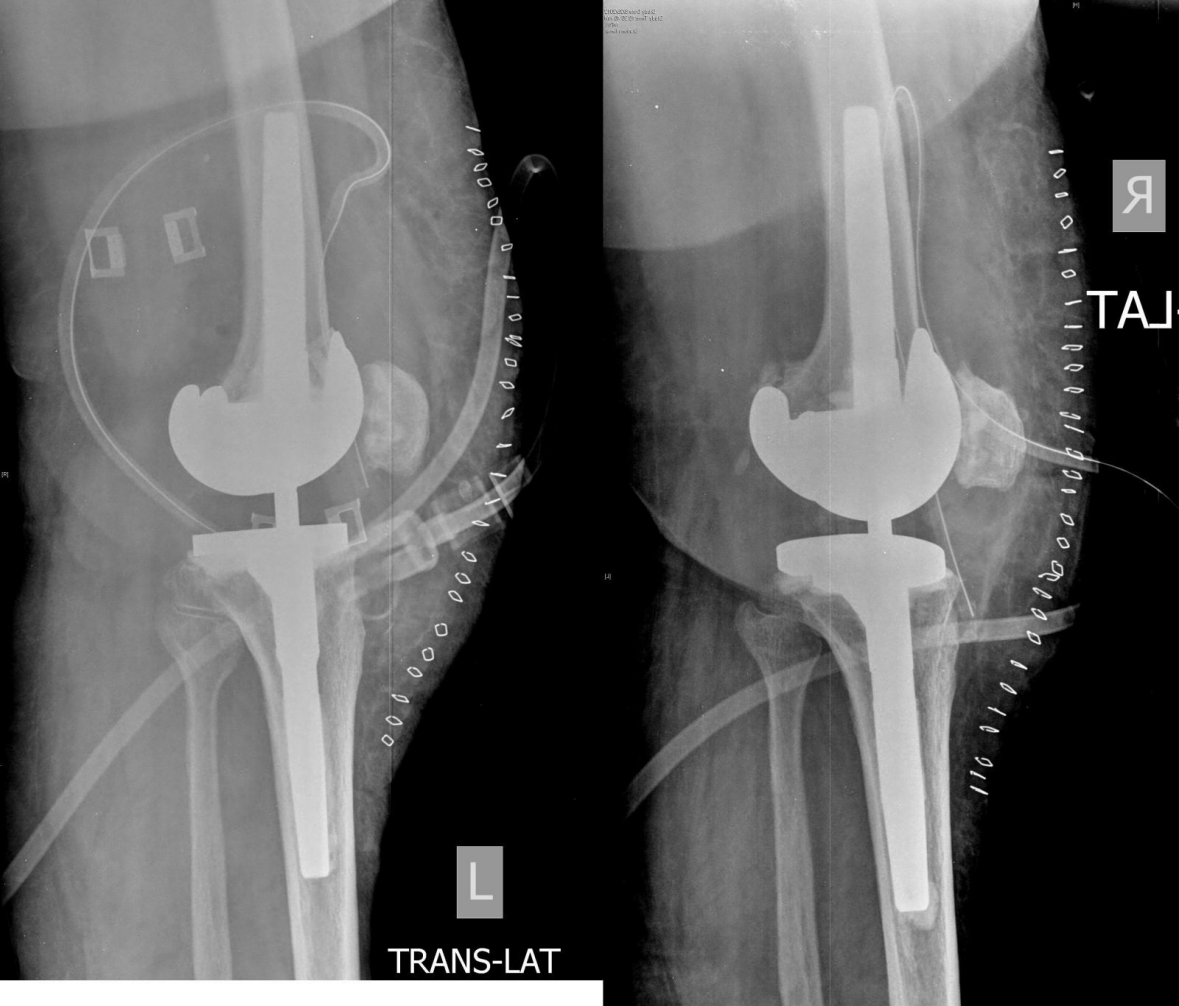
Postoperative lateral radiographs showing well-aligned new constrained implants in both the knees.
